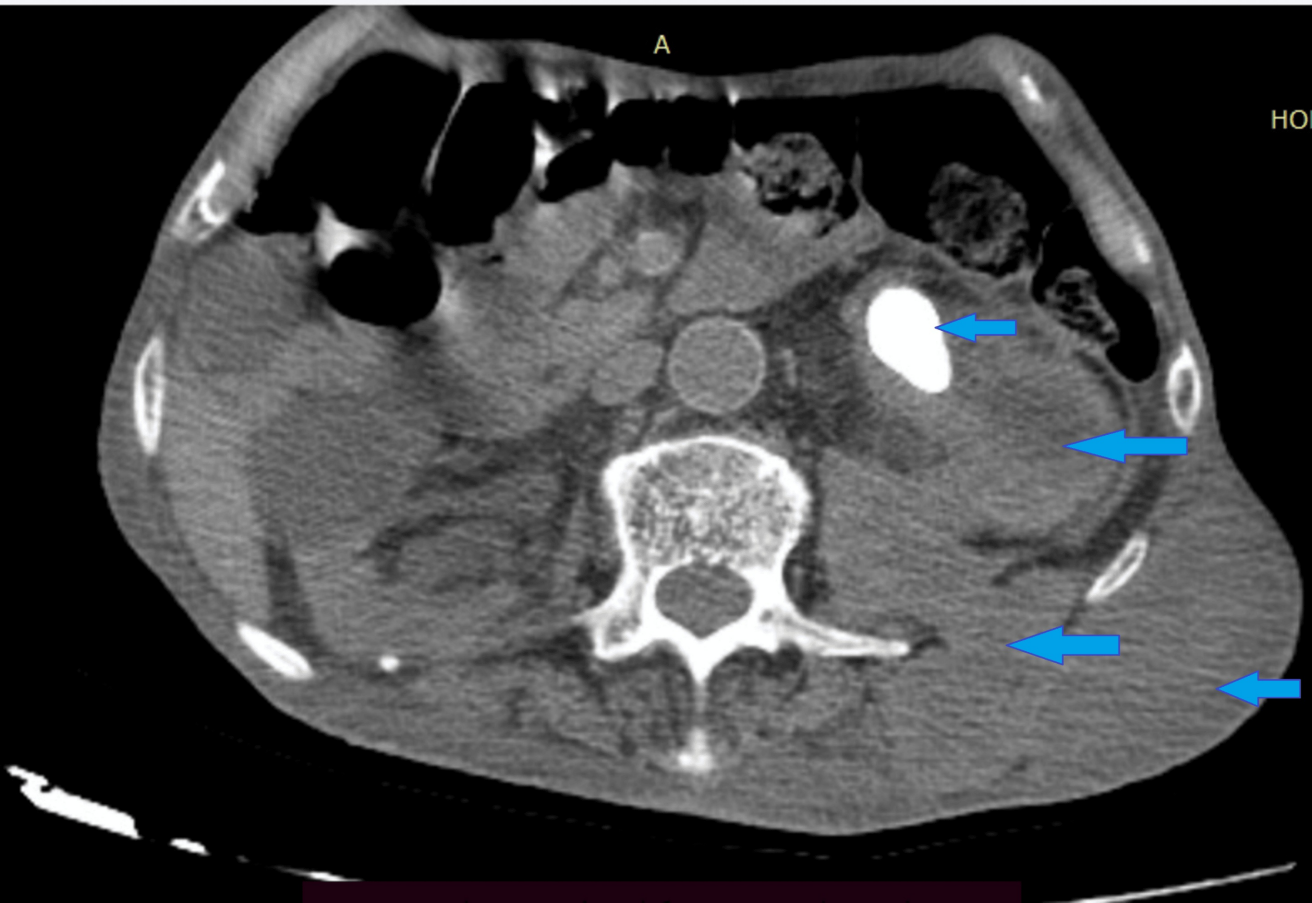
Axial image of an abdominopelvic CT scan The image reveals left pyonephrosis caused by an obstructive renal pelvic stone, complicated by a retroperitoneal abscess with fistulization, leading to a subcutaneous lumbar collection in the affected region, indicated by blue arrows.
